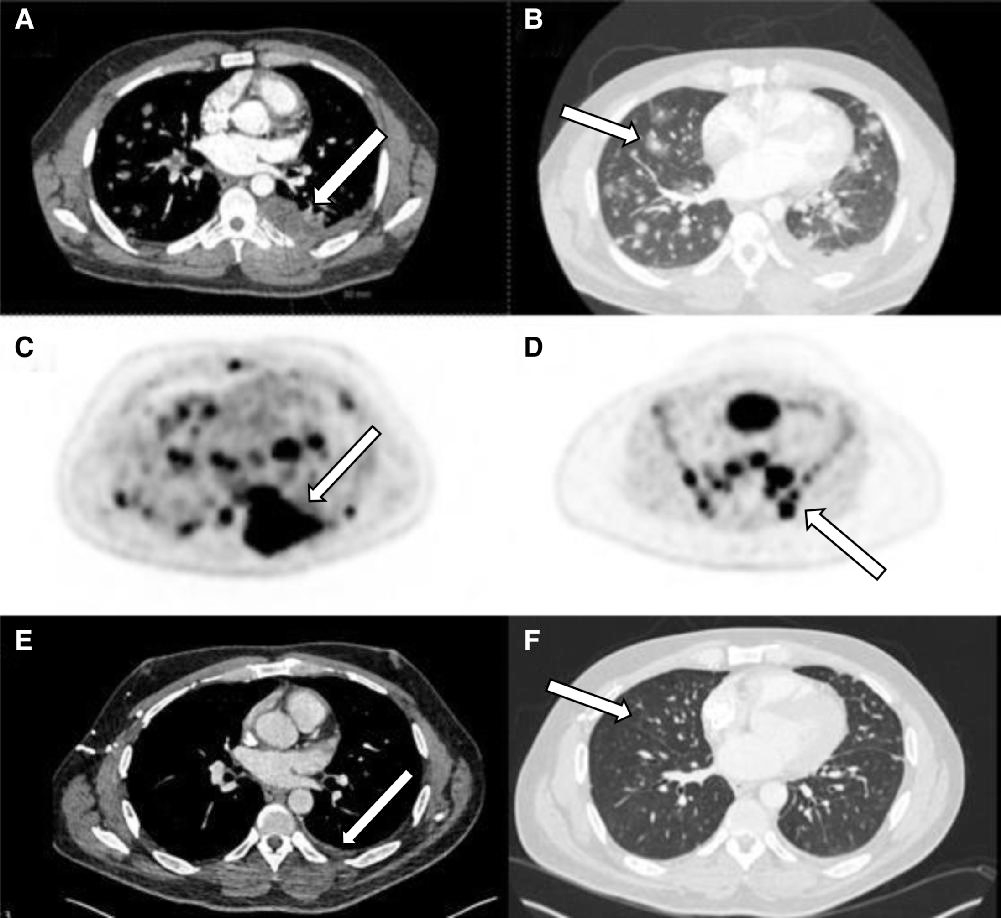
A, Arrow highlights a left pleural based bass, measuring 5.0 × 2.6 × 4.4 cm. B, Numerous lung nodules, some with central cavitation, with some associated ground-glass opacification. C, Arrow highlighting one such nodule. Fluorodeoxyglucose positron emission tomography computed tomography (FDG PET CT) redemonstrated a now enlarged 6.8 × 6.9-cm left pleural-based mass (highlighted by arrow), with an standardized uptake value (SUVmax) of 19.6. D, FDG PET CT also demonstrated extensive FDG-avid osseous lesions. One such representative lesion (arrow) included here on the left ischium with an SUVmax of 15.8. E, Marked improvement in size of pleural mass (arrow) and F, lung nodules compared to prior images 8 weeks after selpercatinib (arrow highlighting area of improvement of a lung nodule).
